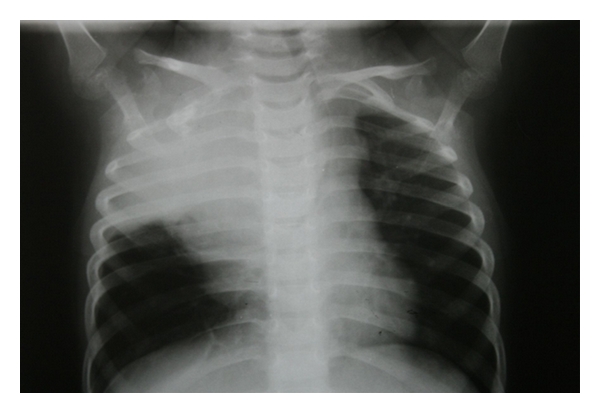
Chest X-ray of Case 1 at the presentation.
